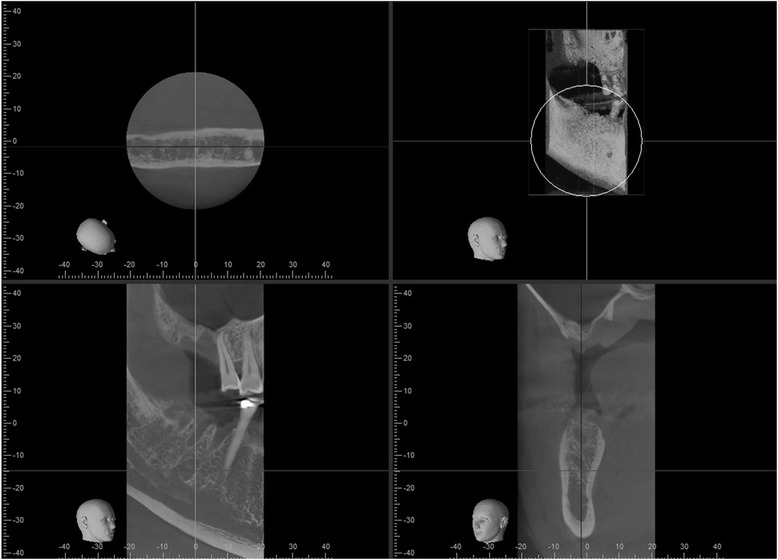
Right sinus prior to first sinus grafting procedure. Cone beam CT imaging shows very little residual bone volume at implant site for the no. 3 area
